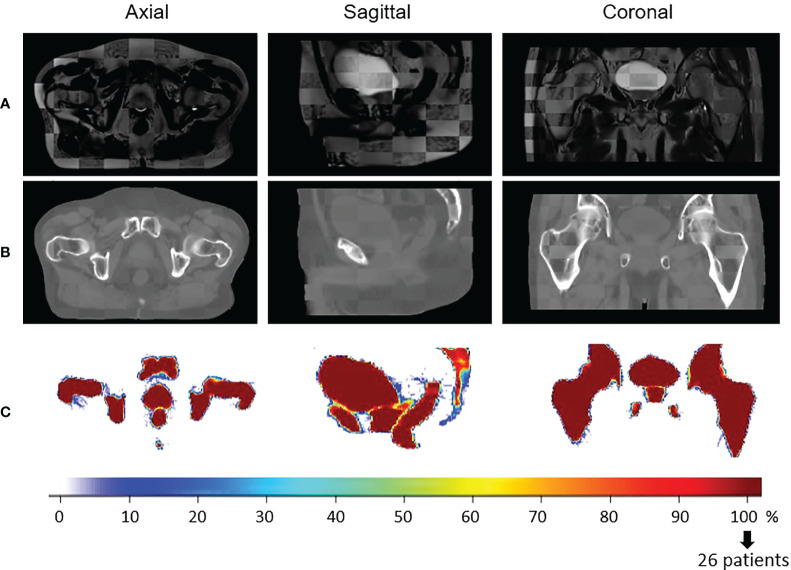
Visual quality control of the interpatient registration. Checkerboard comparison of (A) the template MRI with the mean of all the population MRIs registered in the Common coordinate system (CCS) and (B) the template CT with the mean population CTs in the CCS. Probability maps are presented in (C). It is the result of the overlapping of all the delineated structures in the same space to estimate the precision of the registration. In blue, few structures are overlaid (poor quality of registration). In red, all the patient structures correspond to the same anatomical location (100%, perfect registration).
